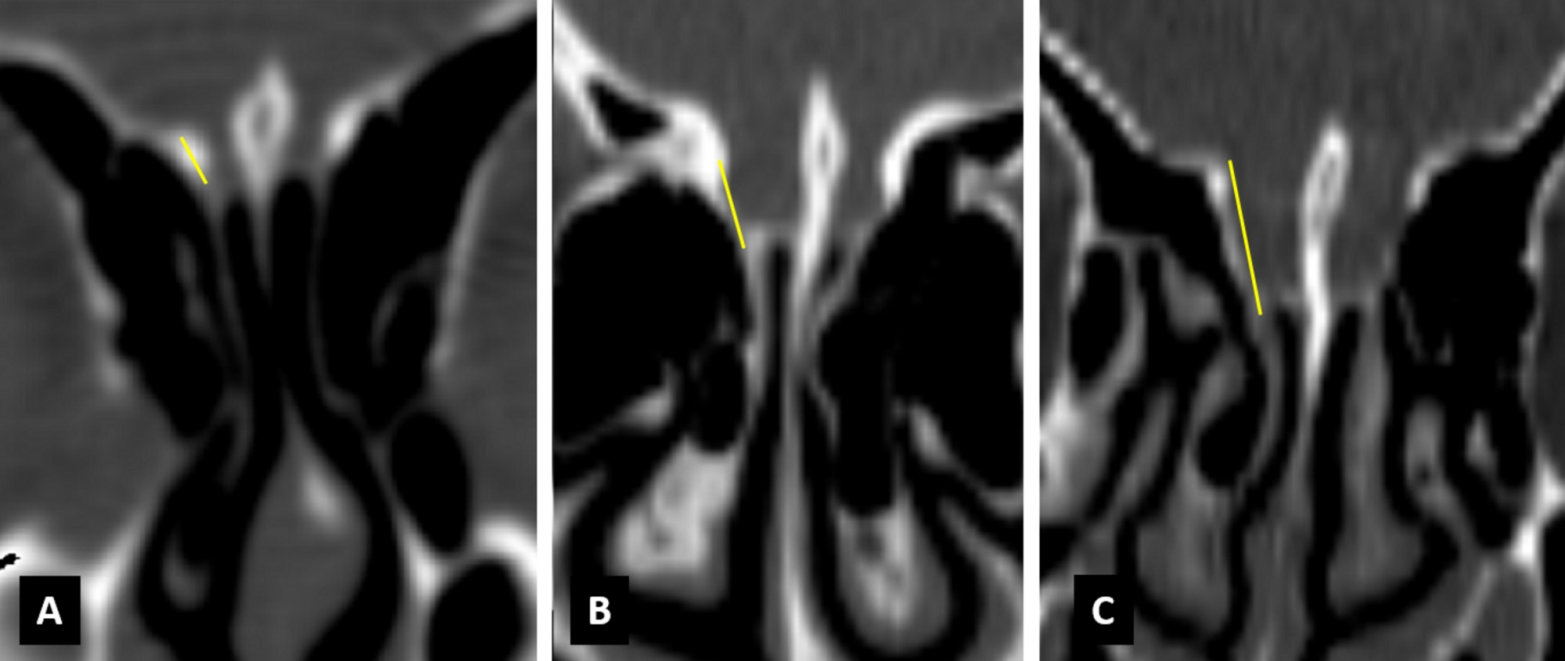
Coronal CT images showing Keros classification Keros (A) type I bilaterally, (B) type II bilaterally, and (C) type III bilaterally.
